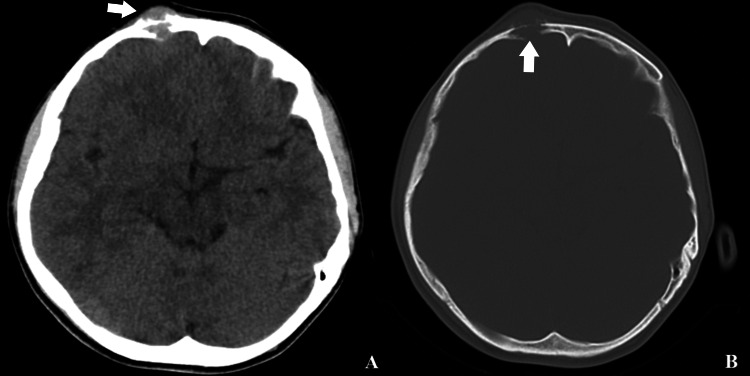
Preoperative computed tomography of the brain showing parenchymal (A) and bone window (B) view of the lesion that erodes the bone and gives an expansile appearance to the skin (White arrow)
